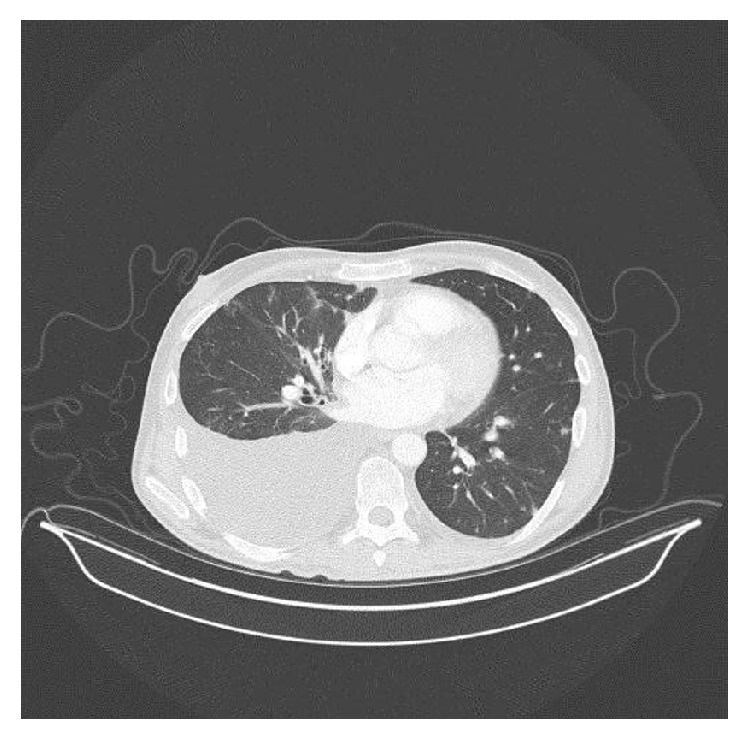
CT examination confirmed a unilateral right pleural effusion and small lung nodules (transverse computed tomographic image of patient's chest demonstrating posterior right chest effusion).
